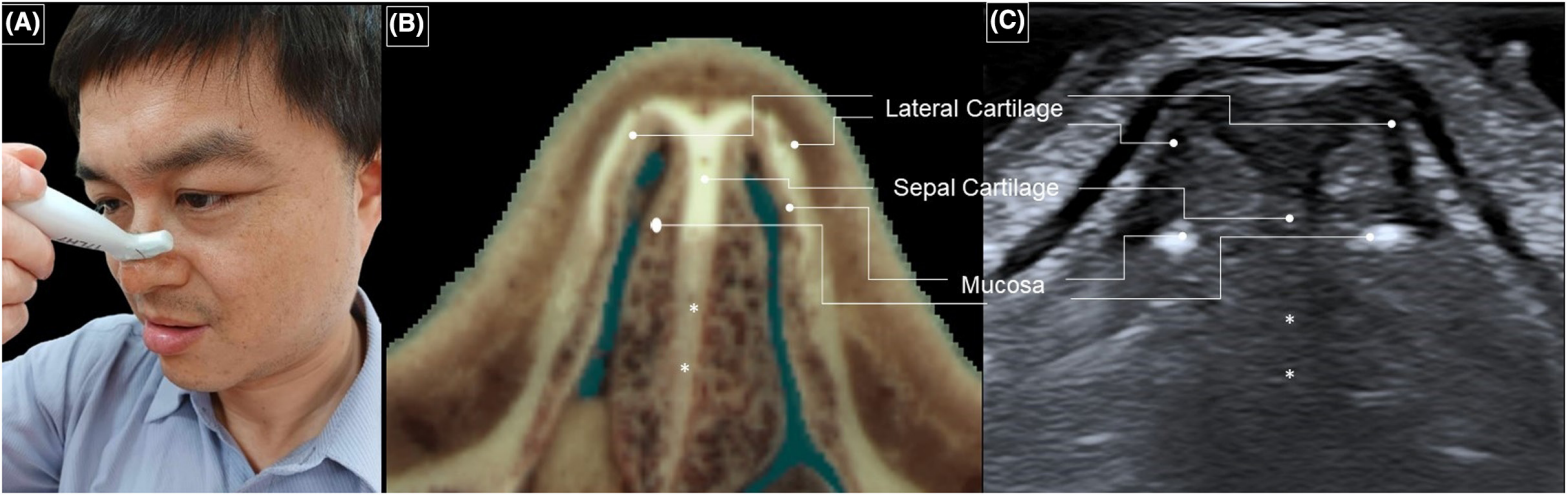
(A) The hockey‐stick transducer is positioned over the nasal bridge near the nasal tip in the horizontal plane. (B) The cadaveric cross‐sectional image and (C) the corresponding ultrasound scan show the nasal cartilage. Note that the bottom part of the nasal cartilage (*asterisk*) is barely visible in this maneuver. Cadaveric images are adapted from the Visible Human Project of the National Library of Medicine. Excerpts from these images, featured in the VH Dissector, are used with permission from Touch of Life Technologies Inc. Images showing the transducer position on our corresponding author's face are also used with permission for publication.
